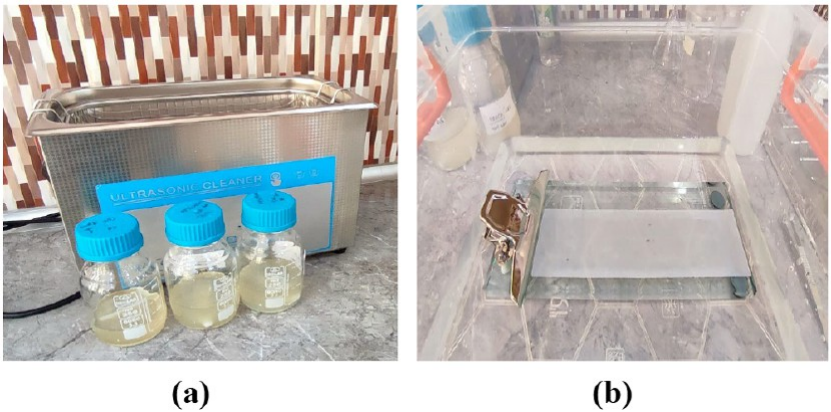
Ultrasonic water bath (a) and phase inversion in the water
